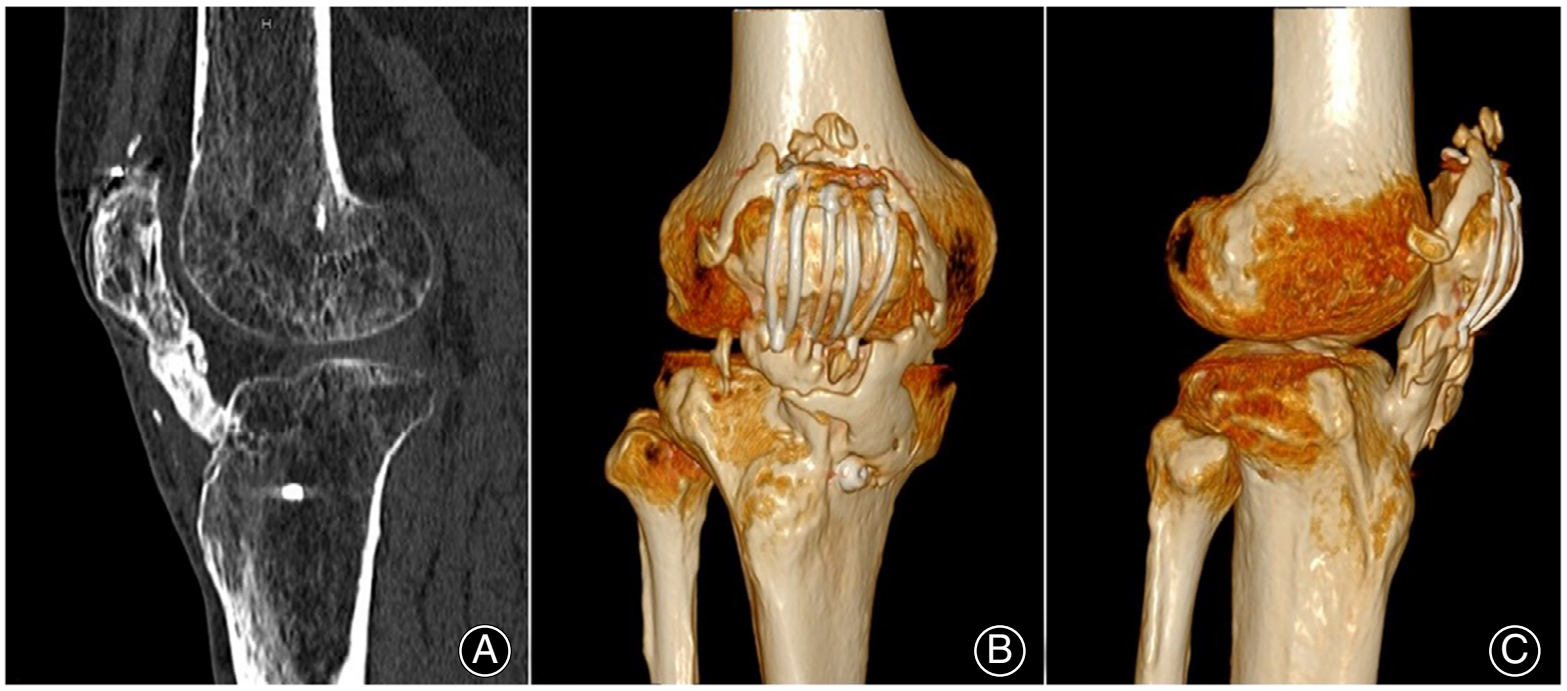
Sagittal CT scan image (A) and three‐dimensional reconstruction CT images (B, C) of the patient's right knee show extensive patellar tendon ossification throughout its whole length.
